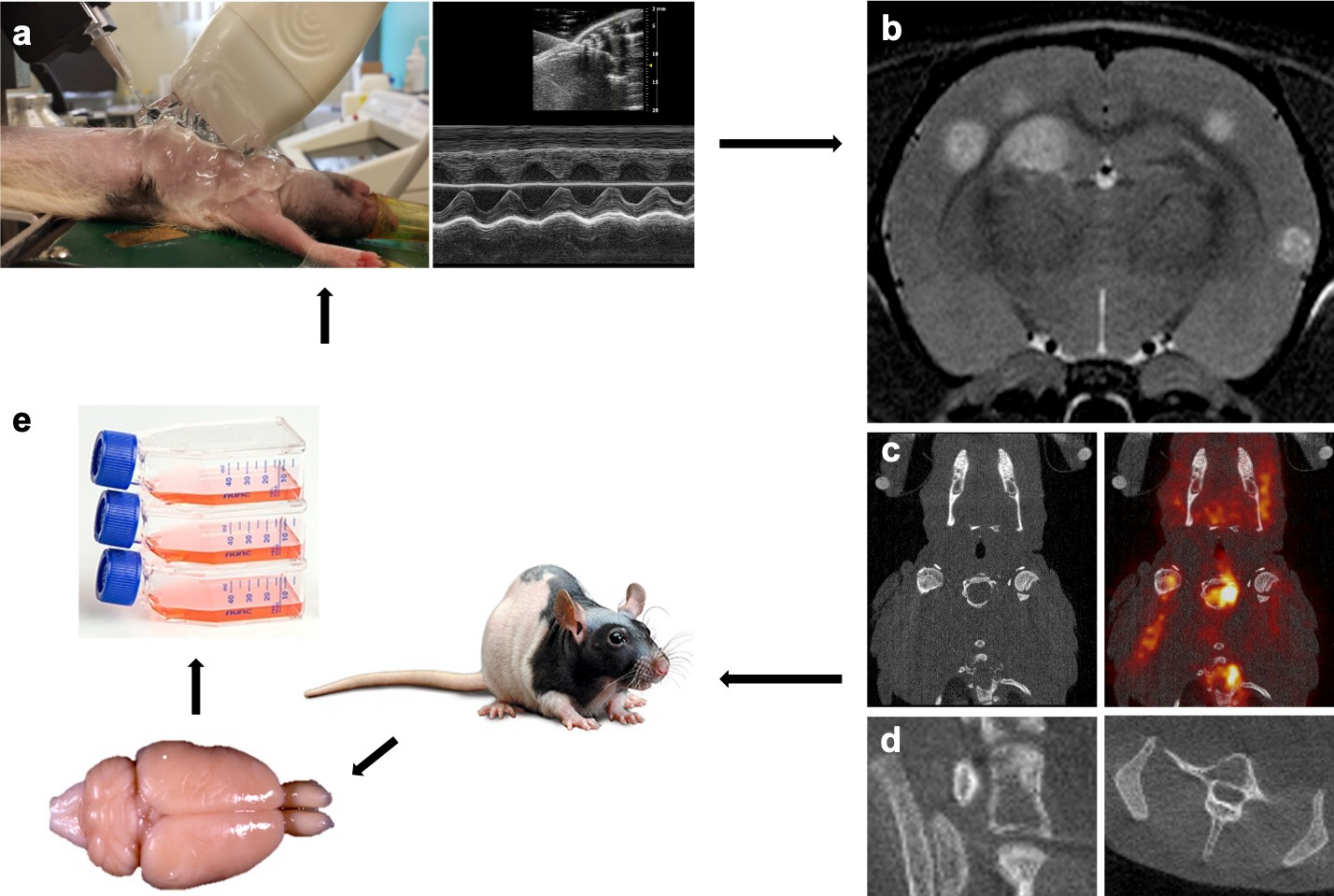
Establishment of the MDA-MB-231br/eGFP subpopulations. a. Intracardiac injection of the MDA-MB-231br/eGFP cancer cells using ultrasound guidance. b-d. Follow-up of metastasis development with multimodal imaging. Preclinical 7 T MRI for assessment of brain metastases (b), [^18^F]FDG PET/CT for visualization of extracranial metastases (c), and high-resolution CT for the detection of bone metastases (d). e. Isolation of the MDA-MB-231br/eGFP cancer cells from the brain metastatic lesions followed by cell culture. This procedure was repeated five times.
